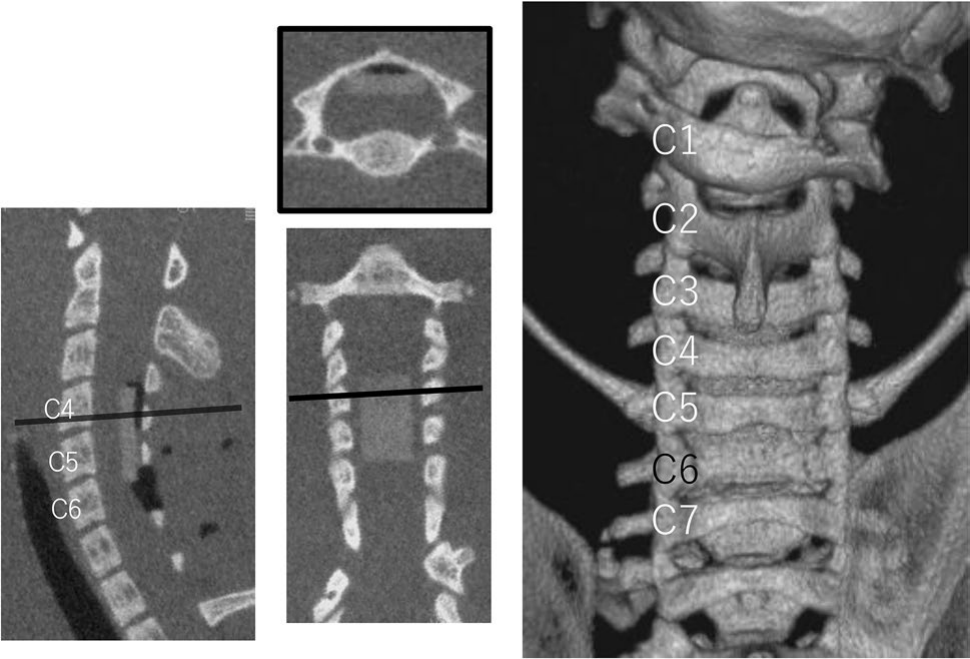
A CT taken just after the sheet insertion surgery. A C6 laminectomy was performed and a sheet was inserted on the dorsal spinal cord at the C4–C5 level.
